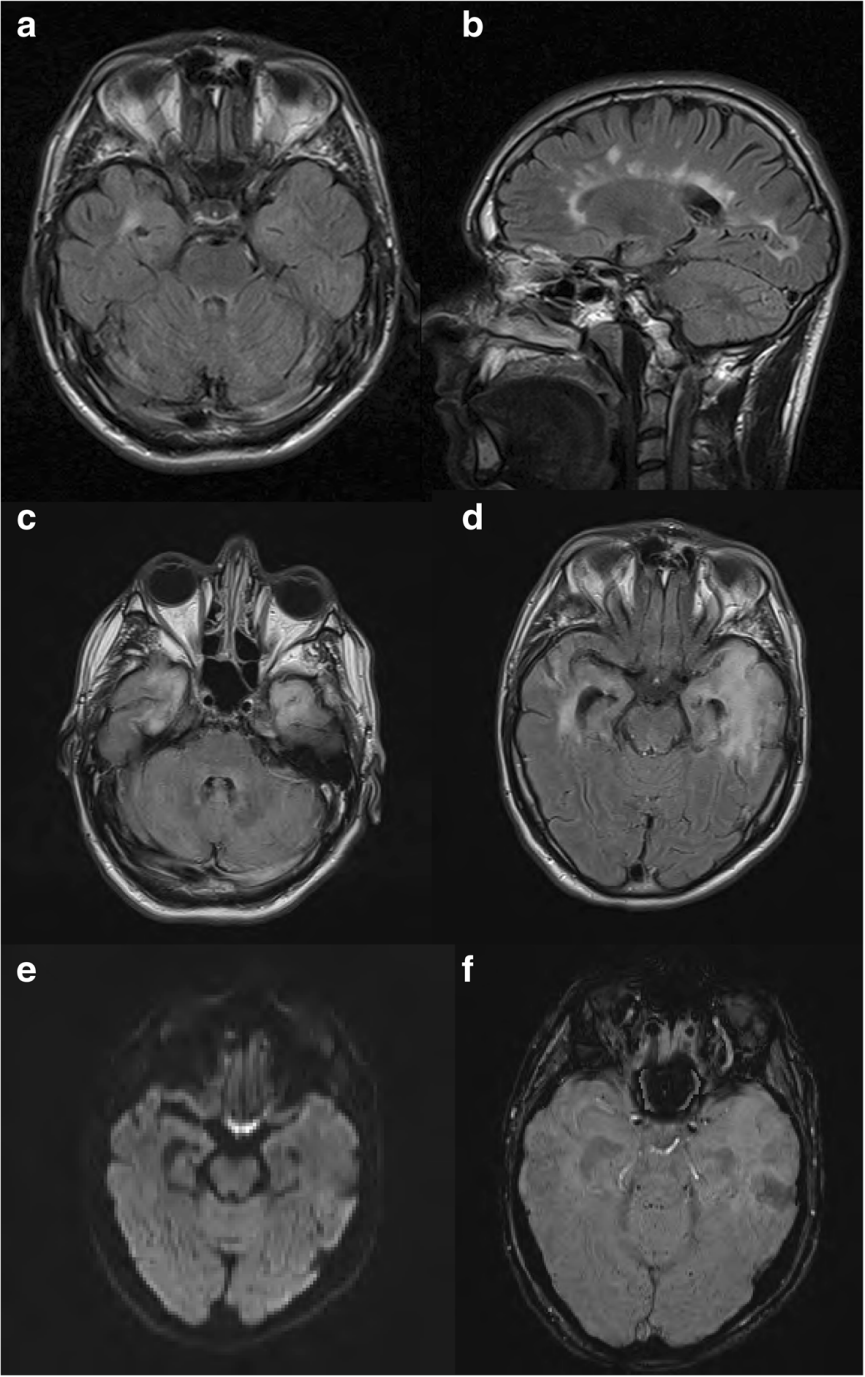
Axial FLAIR (a) and saggital FLAIR (b) sequences from last surveillance MRI for his MS taken 2 months prior to presentation shows stable periventricular white matter lesions with evidence of an isolated demyelinating plaque in his right temporal horn. Axial FLAIR (c and d) sequence at presentation shows high signal abnormality in both temporal lobes extending to the insular cortex. Axial diffusion weighted imaging (e) shows no restricted diffusion and susceptibility weighted imaging (f) shows no area of focal haemorrhage
